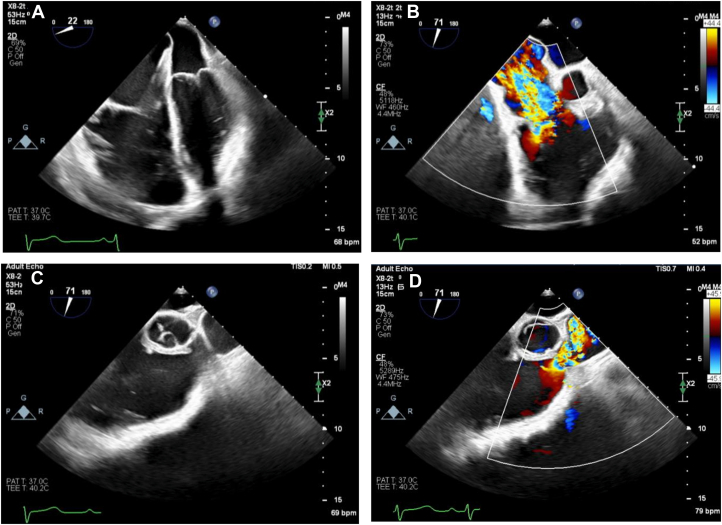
Baseline TOE Imaging at the Time of Cardiac Surgery (A) Grossly dilated right ventricle and right atrium with thickening of the tricuspid valve. Normal-size LV and LA seen. (B) Severe TR seen on baseline intraoperative TOE. (C) RVOT on intraoperative TOE. (D) Demonstration of mild pulmonary stenosis and pulmonary valve thickening on intraoperative TOE. LA = left atria; LV = left ventricle; RVOT = right ventricular outflow tract; TOE = transesophageal echocardiography; TR = tricuspid regurgitation.
